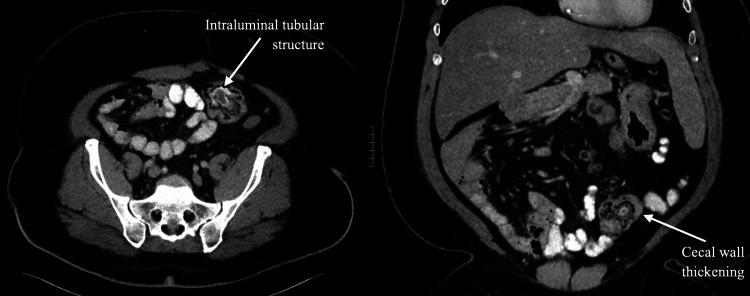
The CT of the abdomen and pelvis demonstrating peripherally calcified 4.5 cm tubular structure protruding into the cecal lumen with cecal wall thickening. Notably, the cecum is found in the left lower quadrant.
